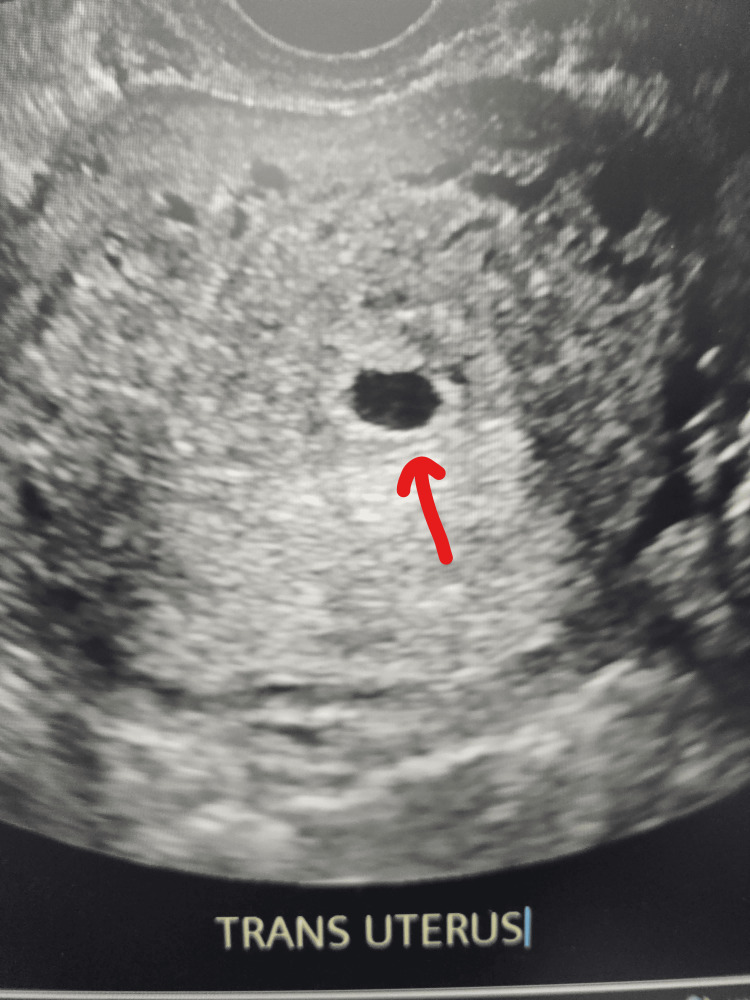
Ultrasonogram showing early intrauterine pregnancy (arrow)
